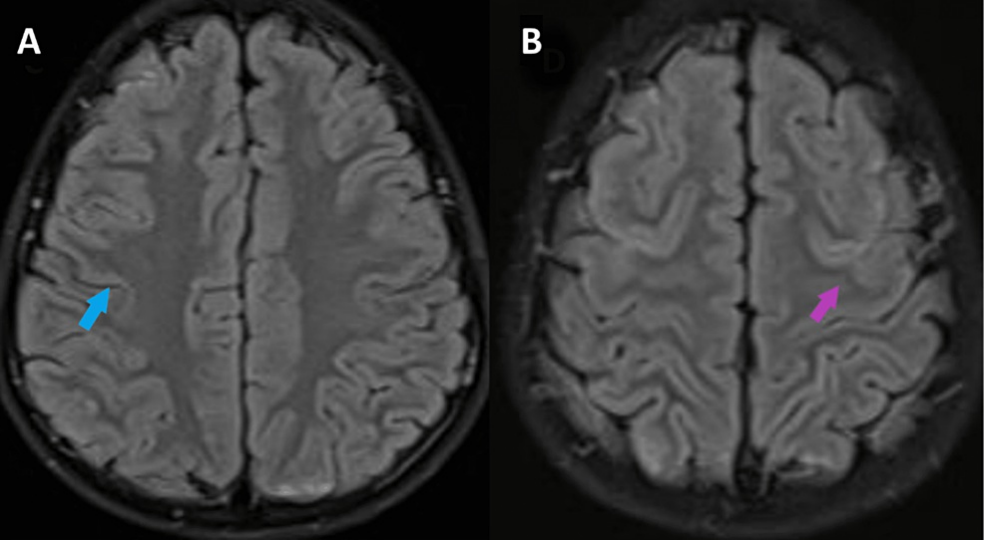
Repeat brain magnetic resonance imaging-FLAIR sequence three weeks after presentation Repeat brain MRI-FLAIR sequence showing resolution of hyperintensities noted on initial imaging (A-blue arrow, B-violet arrow) FLAIR: fluid-attenuated inversion recovery
